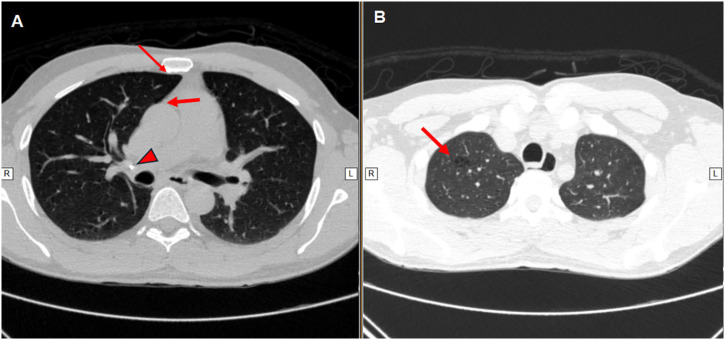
(A) Chest CT showing a calcified nodule in the right hilum (arrowhead), paraseptal emphysema (arrows), and bronchiectasis in the anterior segment of the right upper lobe; (B) Centrilobular emphysema in the apical segment of the right upper lobe and esophageal dilation.
